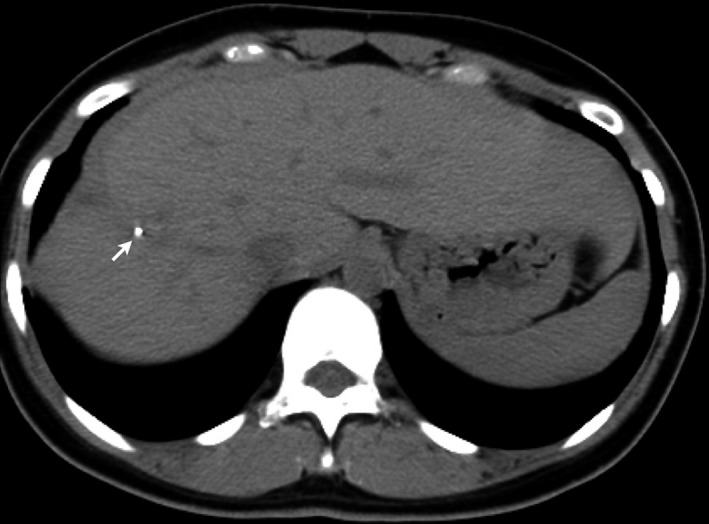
Follow‐up computed tomography in a 19‐year‐old woman 1 year after a high‐grade hepatic injury caused hemodynamic instability. Arrow indicates injection site of n‐butyl cyanoacrylate.
